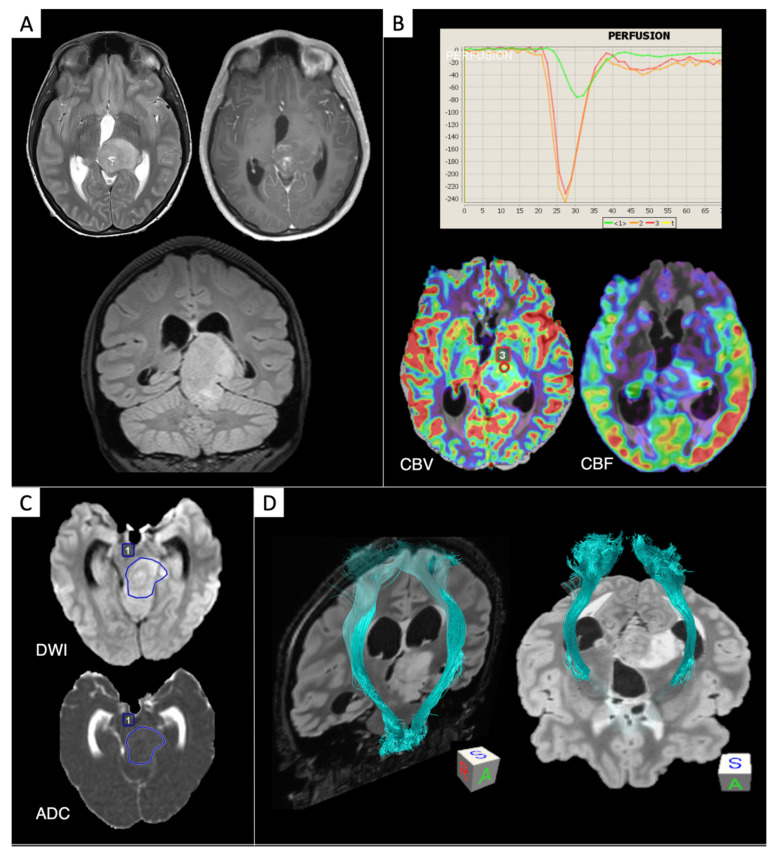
Diffuse midline glioma H3K27M^mut^. MRI datasets were retrieved from the archive of our Institution. (A) Conventional MRI (cMRI): T2, contrast-enhanced T1, FLAIR (coronal section). (B) PWI: dynamic susceptibility contrast (DSC) curve (red and orange: tumor ROIs; green: NAWM), DSC-cerebral blood volume (CBV) (red: tumor ROI), arterial spin labeling (ASL)-cerebral blood flow (CBF). DSC “hotspot” ROI evaluation revealed “aggressive” perfusion features (red ROI: CBV_max_, 4 mL/100 g; CBV_max_/CBV_NAWM_, 6.48); ASL-CBF showed highly perfused spots within the tumor tissue. (C) dMRI: a clinically feasible single-slice ROI evaluation revealed low diffusion parameters (blue ROI: ADC_mean_, 0.87 mm^2^/s; ADC_mean_/ADC_NAWM_ ratio, 1.19). (D) Q-ball tractography of corticospinal tracts, exhibiting a mild ventrolateral dislocation of the left tract due to the mass effect of the lesion.
